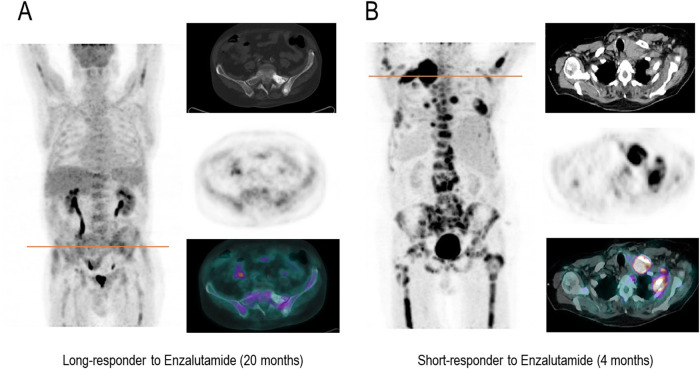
Emblematic cases of divergent response duration to the same systemic treatment in the presence of opposite TLG. In these two patients, FDG PET/CT showed a remarkable mismatch in the extent of the metabolically active metastatic burden (TLG = 59, and TLG = 5602.4 in Panels A and B, respectively), which corresponded to divergent response duration to the same systemic treatment (20 and 4 months after the first dose of Enzalutamide, respectively). On the left side of each panel Maximum intensity projection (MIP) PET images are represented, while on the right side, the axial section of CT, PET and PET/CT images of the hottest metastatic lesions (also indicated by the orange line on the MIP images) are represented from top to bottom, respectively.
